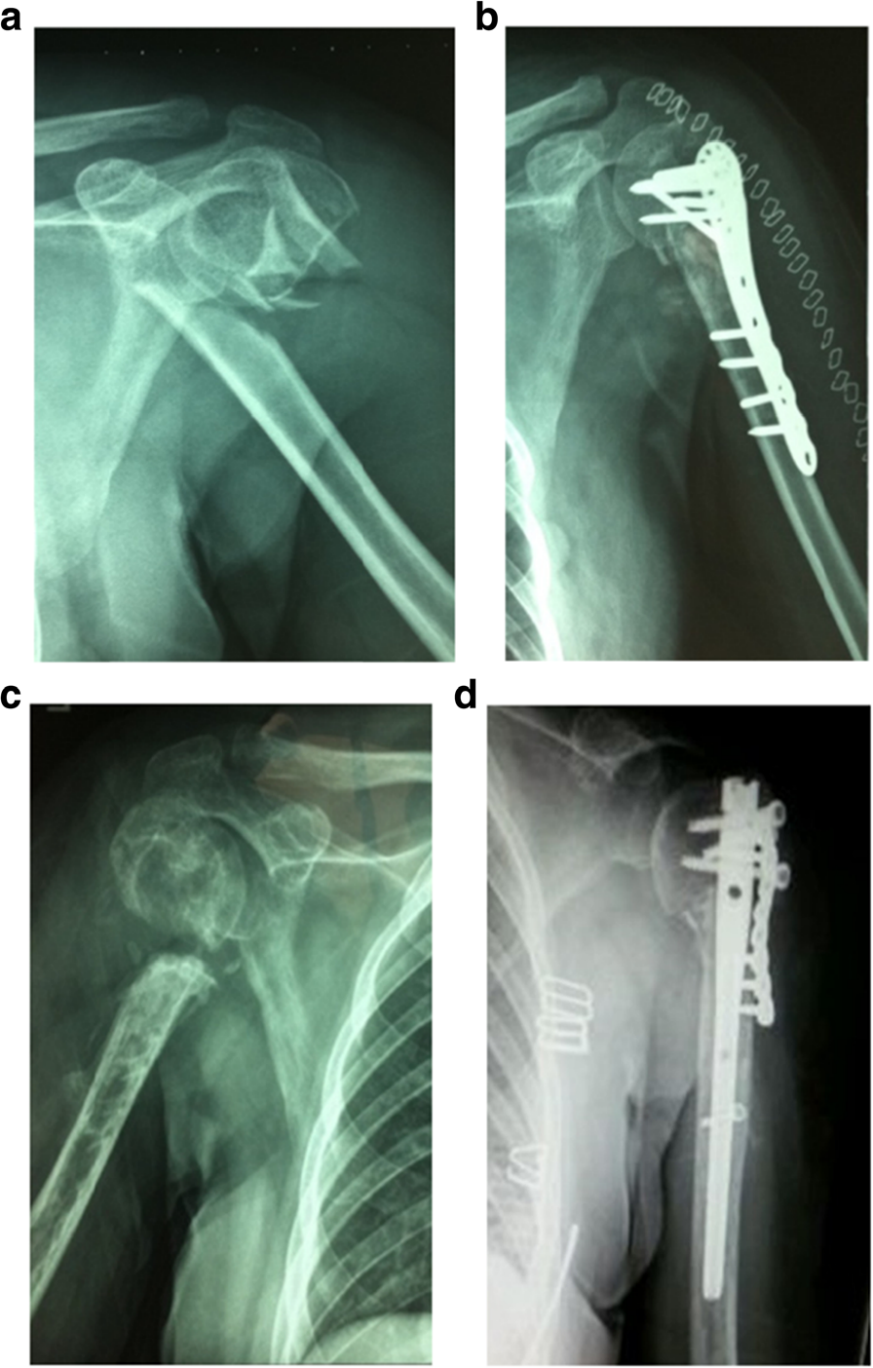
Stable hardware + bone not healed Cierny–Mader types 3 and 4 = remove hardware, temporary stabilize, and reconstruction when clean. a Radiograph of humerus fracture. b Radiographs of primary internal fixation. c The implant was removed with space technique. d Reconstruction with locking intramedullary nail and plate when clean
